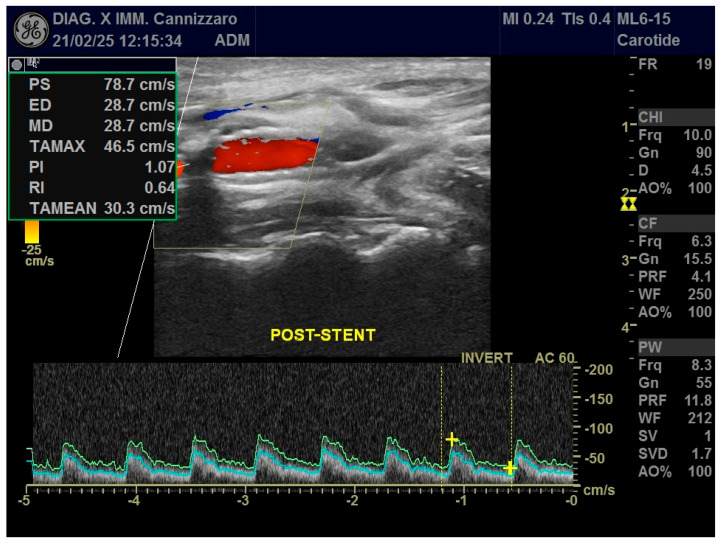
Ultrasound applications in a patient who has received CAS with stent positioning in the right ICA. Color Doppler and Power Doppler modes post-stent show significant hemodynamic stenosis resolution with normalization of VPS values and resistance indices.
